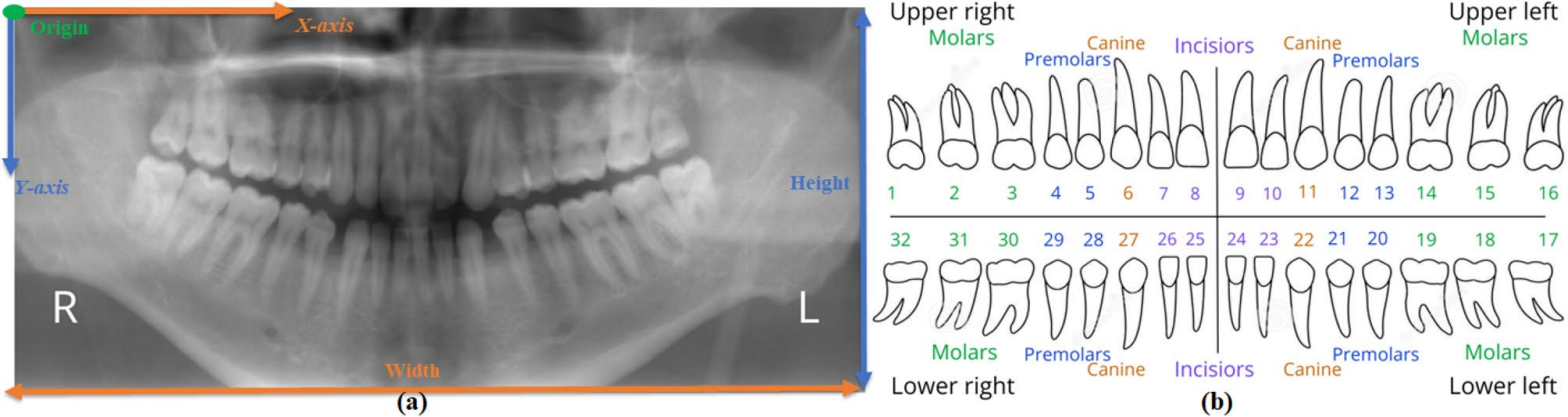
(a) Dental panoramic X-ray image and (b) Universal tooth numbering system.
